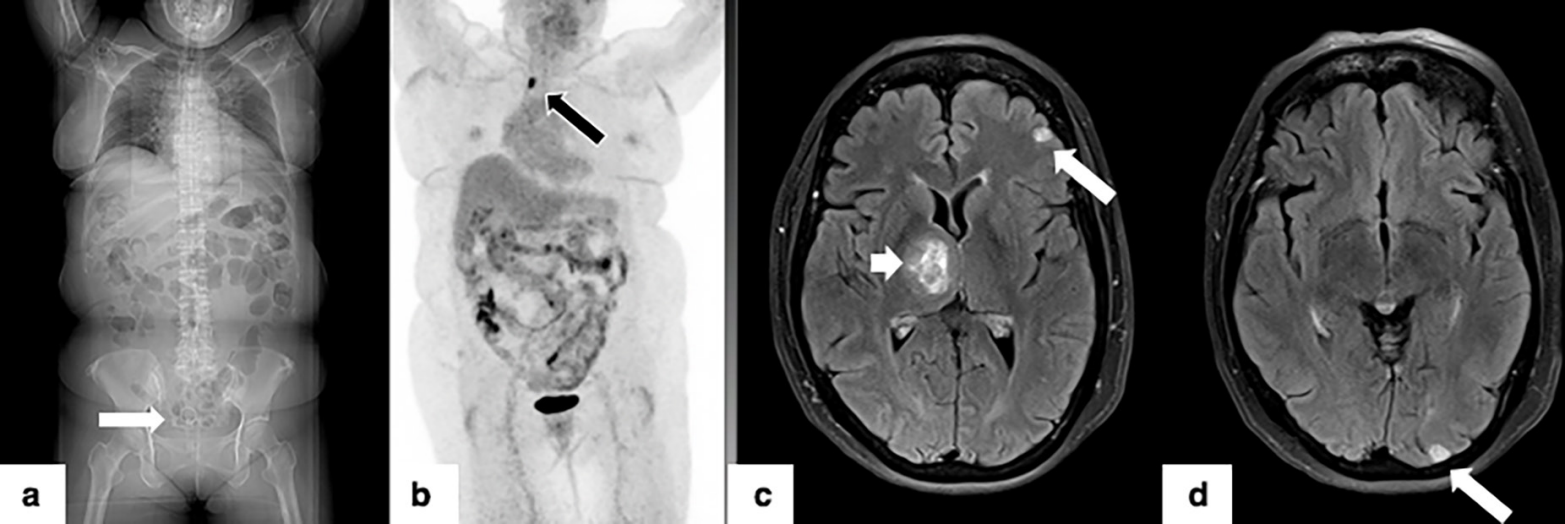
63F with non-small cell lung cancer status post right middle lobectomy and systemic therapy 3 years prior to presentation. Scout images (2a) from PET-CT demonstrate a ring IUD (white arrow) within the pelvis. PET images (2b, black arrow) demonstrate recurrence in the right hilum. Axial post-contrast *T_1_* weighted images of the brain demonstrate multiple metastatic lesions (2c and 2d white arrows) with a dominant mass in the right centrum semiovale and thalamus (2c, small white arrow). The MRI was necessary for pre-operative planning for γ knife therapy.
